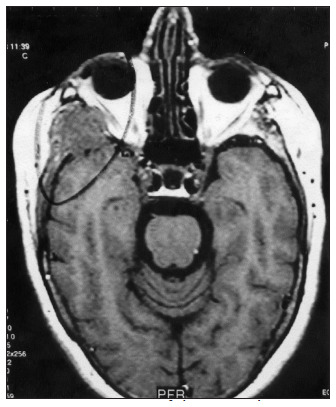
Invasion of the central nervous system by adenoid cystic carcinoma as shown in magnetic resonance imaging.
